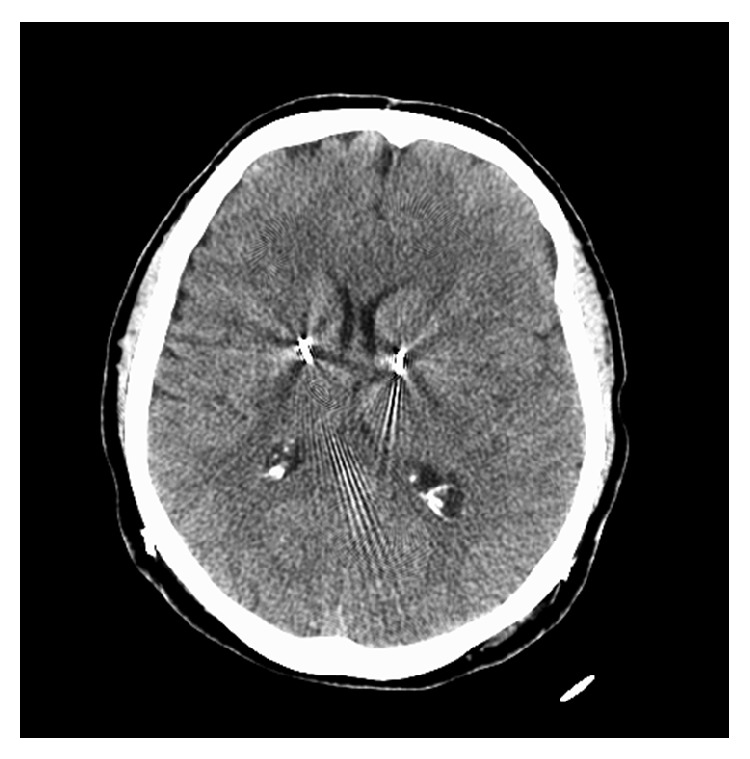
CT head at follow-up visit demonstrates resolution of SDH.
